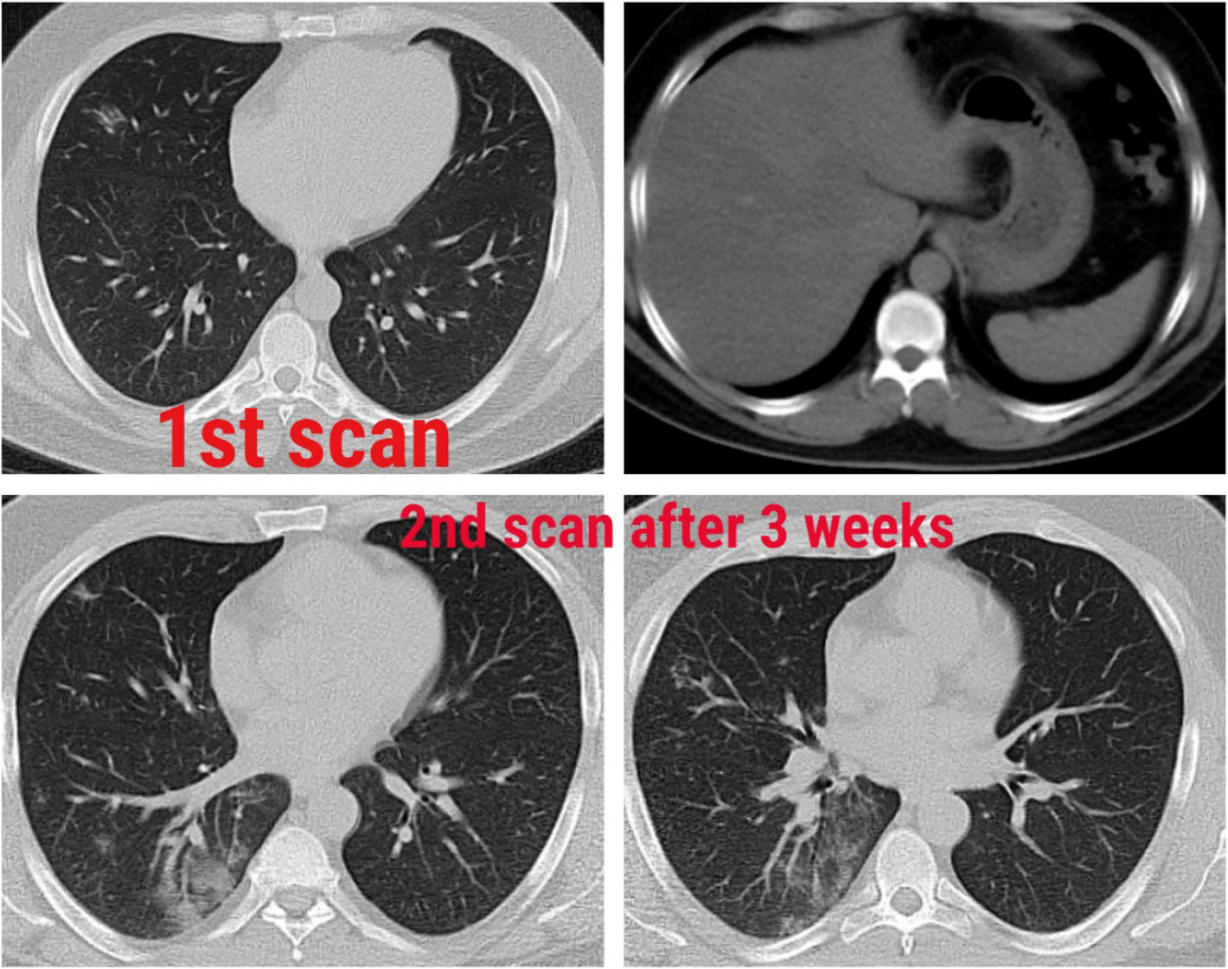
Male patient, 36 years old. The first scan after few days of symptoms shows just a small patchy ground-glass opacity in the middle lobe. The second scan after 3 weeks for follow-up shows a progressive course with multiple pulmonary ground-glass opacities. CT cuts of the upper abdomen show a fatty liver
